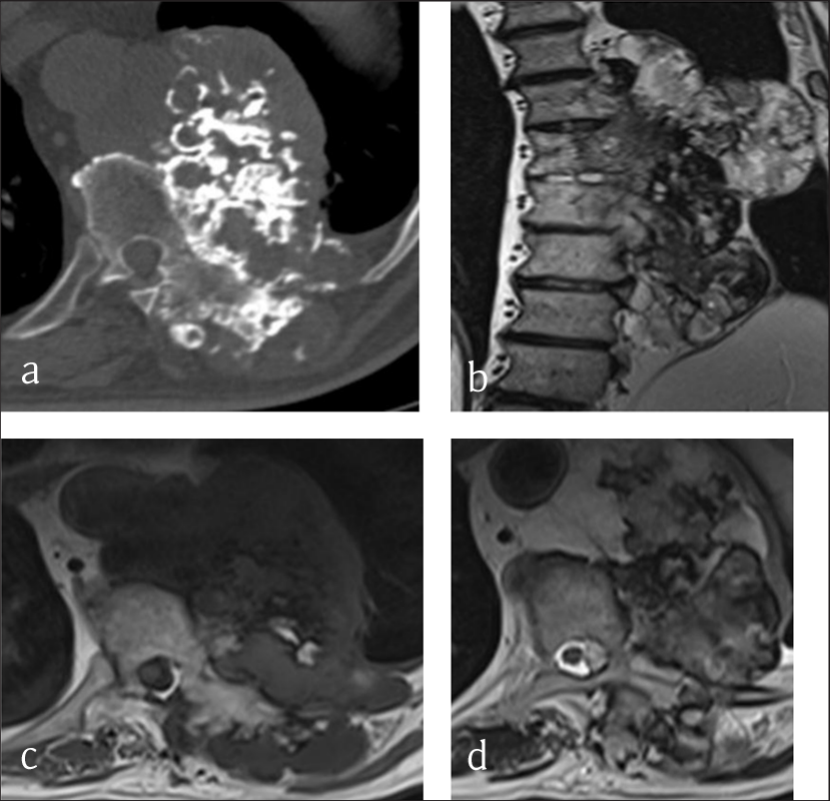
Chondrosarcoma of T8, T9 and T10. (a) Axial CT scan shows a large mass arising from the vertebral body with ring-and-arc calcifications. MRI (b) coronal T2-WI, (c) Axial T1-WI and (d) Axial T2-WI show a heterogenous mass consisting of lobules of intermediate signal intensity on T1-WI with residual intralesional bony trabeculae and high signal on T2-WI sourrounded by hypointense ring-and-arcs.
